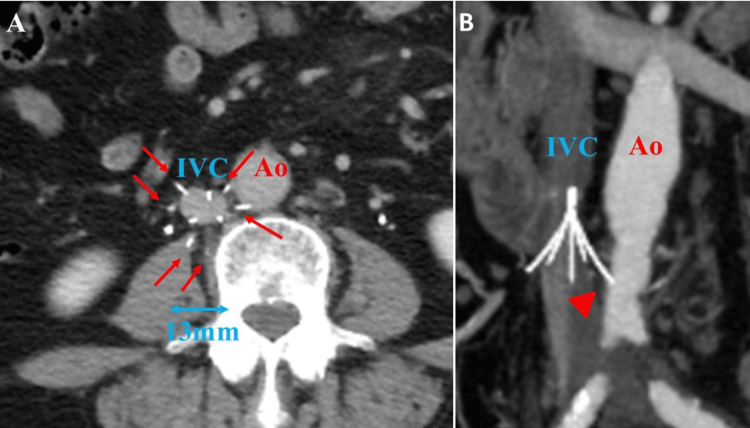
CT findings after 1.5 years of IVC filter placement (A) CT findings 1.5 years after placement of the IVC filter show that the IVC was recanalized and that six of the nine struts of the IVC filter (red arrows) had perforated outside the IVC. The IVC diameter (blue double-headed arrows) is reduced to 13 mm. (B) Struts perforating the left side of the IVC (red arrowhead) were located near the abdominal aorta. Ao: aorta; CT: computed tomography; IVC: inferior vena cava
